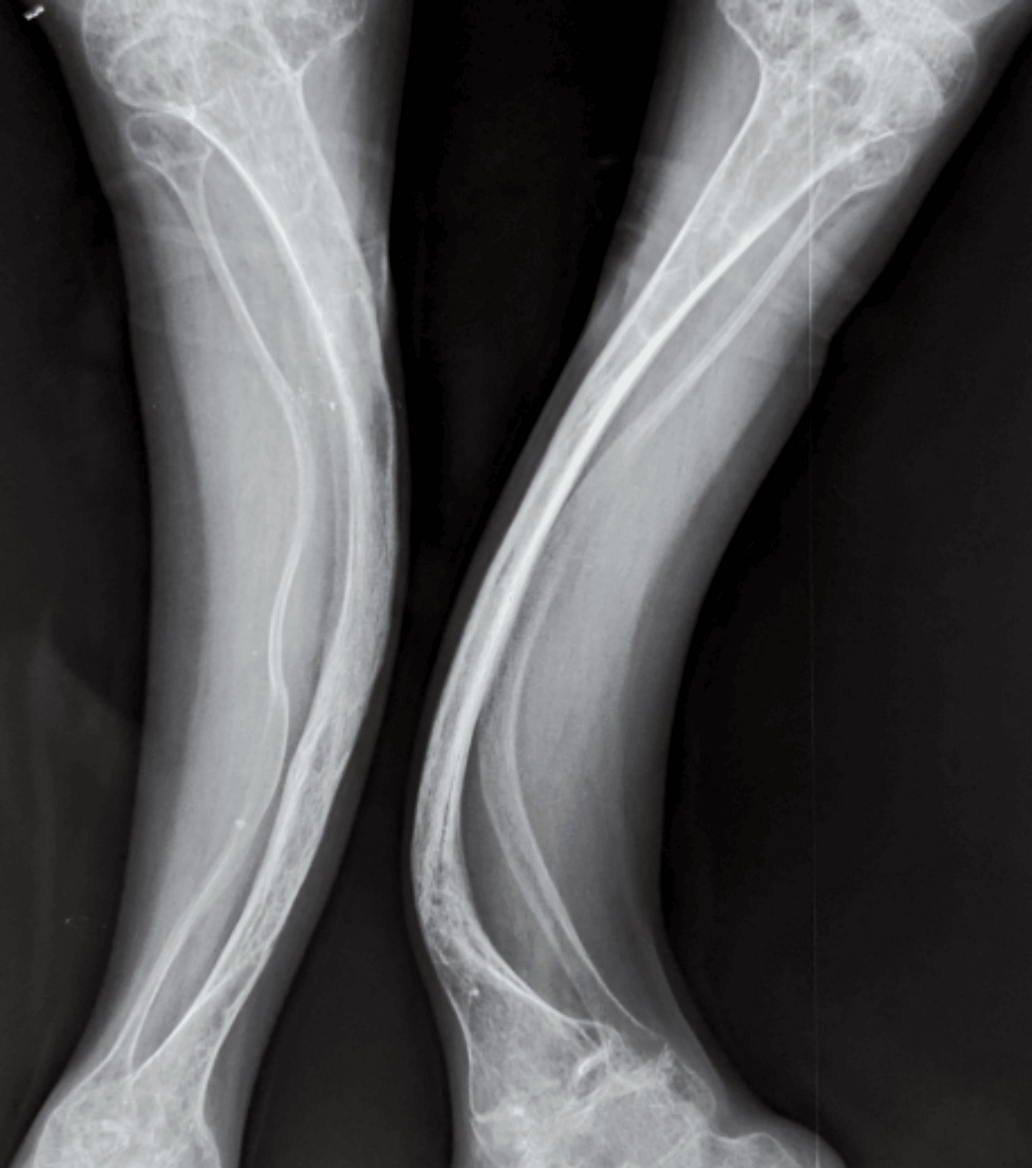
Frontal radiograph of both tibias revealing “saber blade” deformities These bowing deformities are common in children with untreated or advanced OI, reflecting long-standing biomechanical stress on fragile bones. OI: osteogenesis imperfecta
